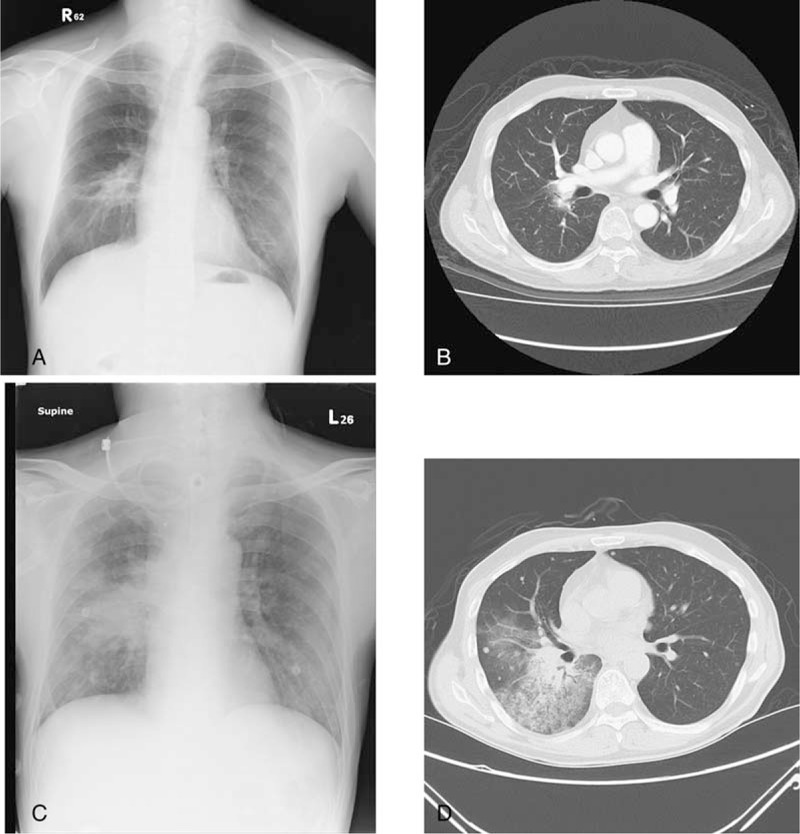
In patient 1, a 54-year-old man with advanced melanoma received a trial of anti-PD-1 treatment with pembrolizumab combined with radiotherapy. Before anti-PD-1 treatment, chest radiograph (CXR) and computed tomography (CT) revealed pulmonary lesions over bilateral hilums (A and B). After 4 cycles of anti-PD-1 treatment, the findings significantly progressed and air-bronchograms at right lower lobe with obstructive pneumonitis was found (C and D). CT = computed tomography, CXR = chest radiograph, PD-1 = programmed cell death protein 1.
